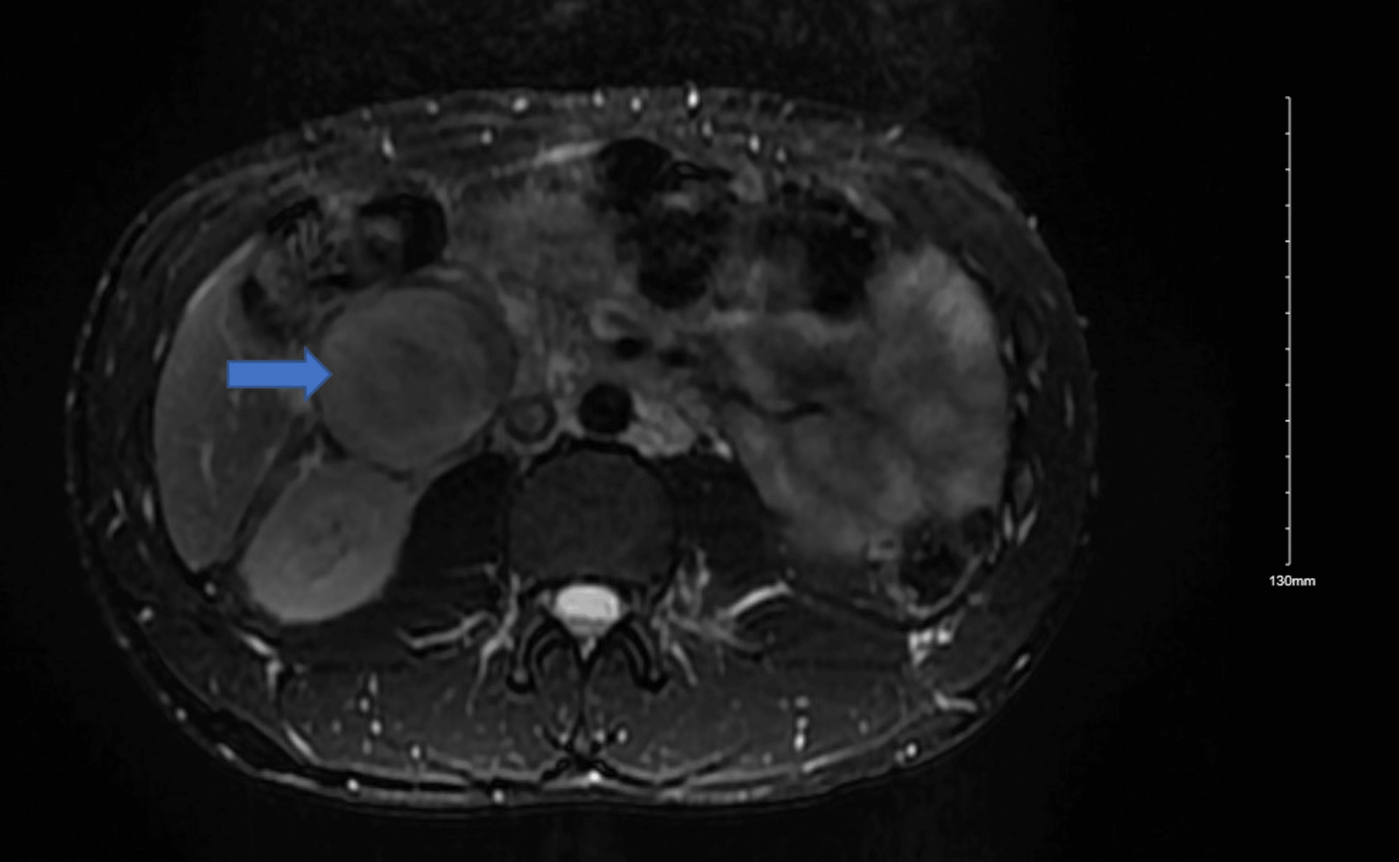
MRI of the abdomen demonstrating a retroperitoneal mass compressing the infrarenal inferior vena cava (blue arrow).
